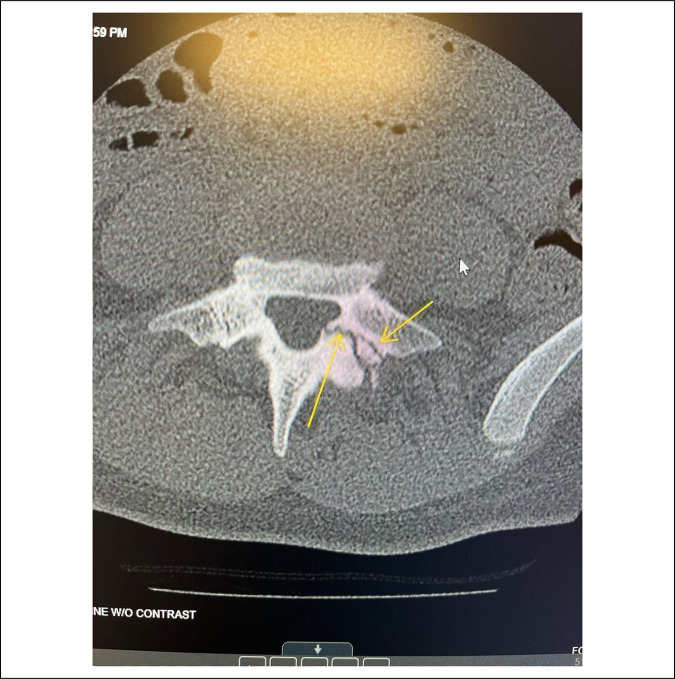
CT scan showing pars fracture at the level of L5 on the left.
